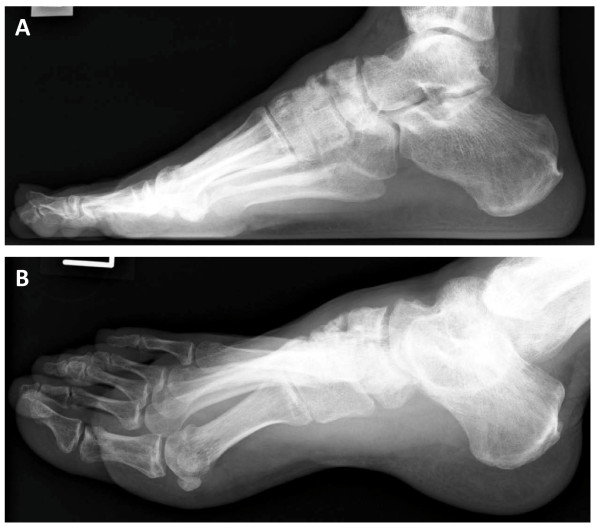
Radiographs at the time of presentation showed atrophic bones and non-union of navicular fragments. (A) Lateral radiograph. (B) Oblique radiograph.
